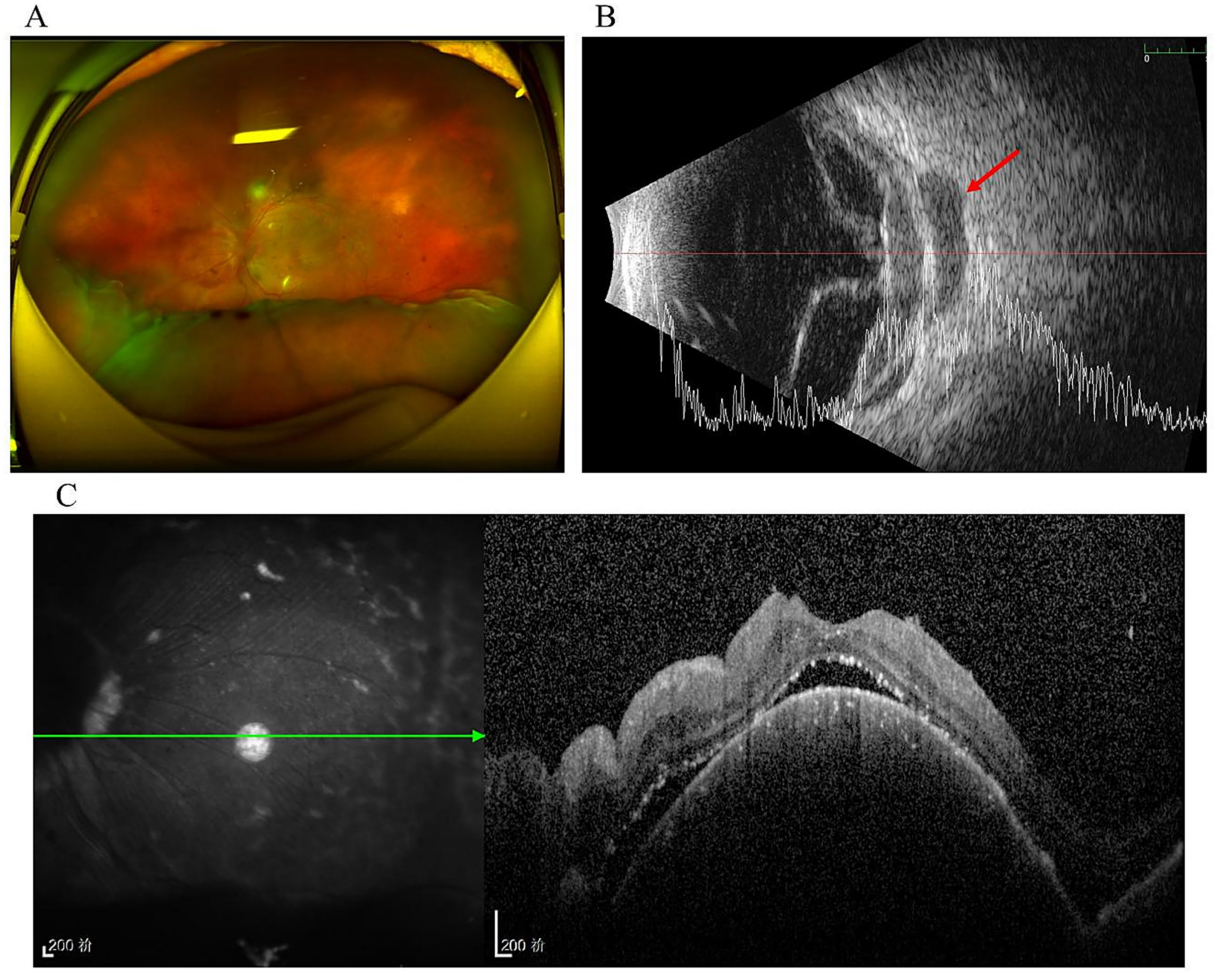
One year after initial presentation. (A) Fundoscopy showed inferior exudative retinal detachment in the left eye; (B) Ultrasonography revealed that retinal detachment, the area of diffuse choroidal low-reflective mass and retrobulbar hypoechoic were significantly enlarged (arrow); (C) OCT of the left eye showed domed choroid thickening and retinal folds.
